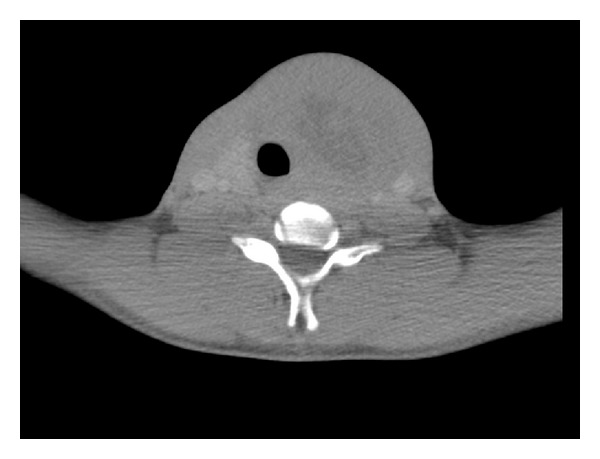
Staging CT showing a large (6.7 cm × 5.8 cm × 6.1 cm) heterogeneous mass in the left lobe and isthmus of the thyroid.
